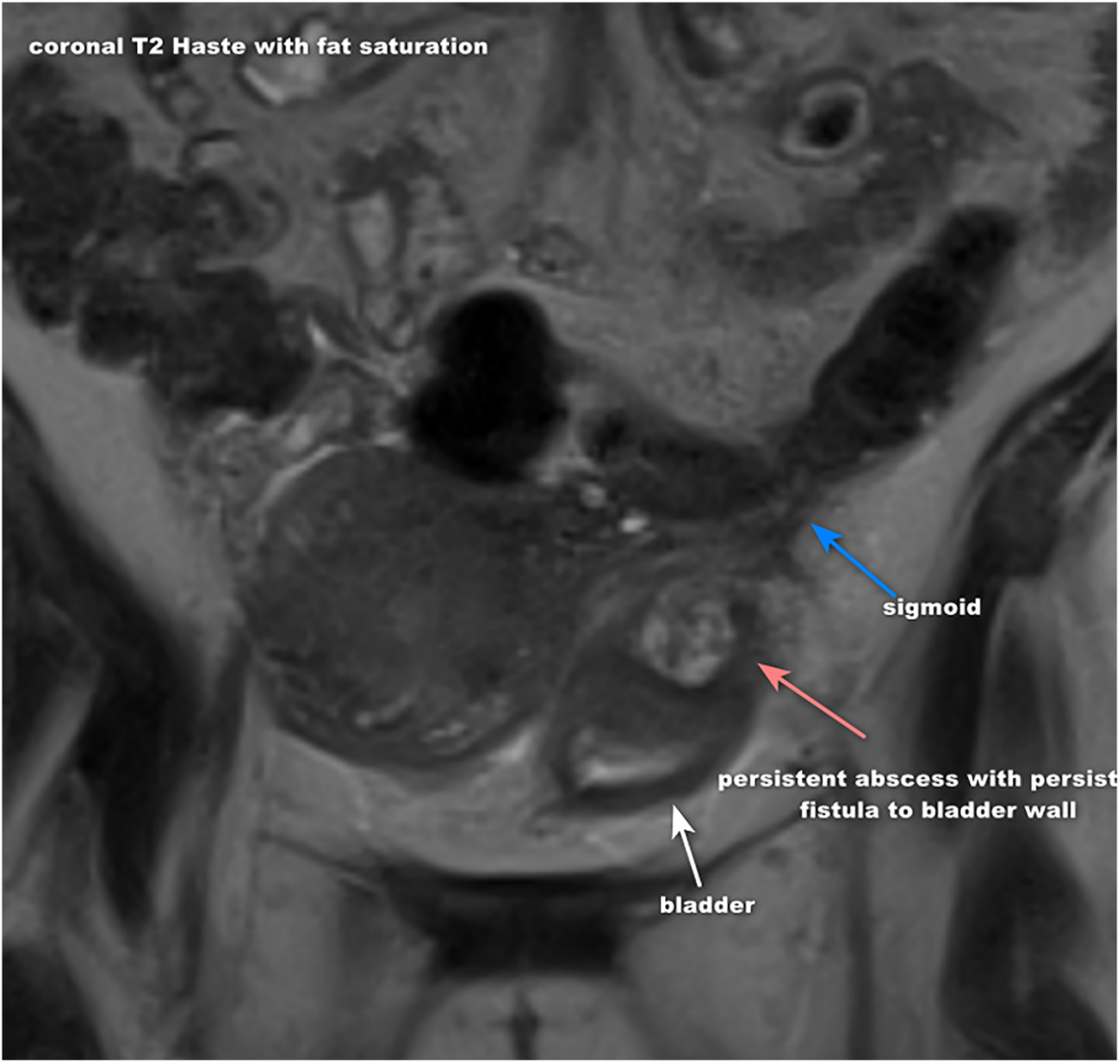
Repeat MRI/abdomen postpartum showing persistent abscess and fistula to the bladder wall.
